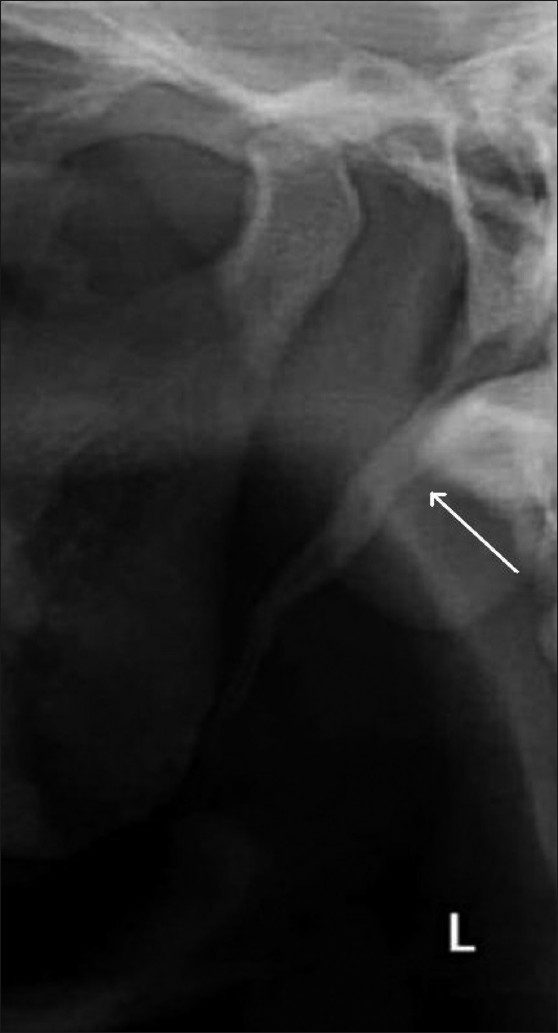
The orthopantomogram shows a Langlais type I styloid process (arrow)
